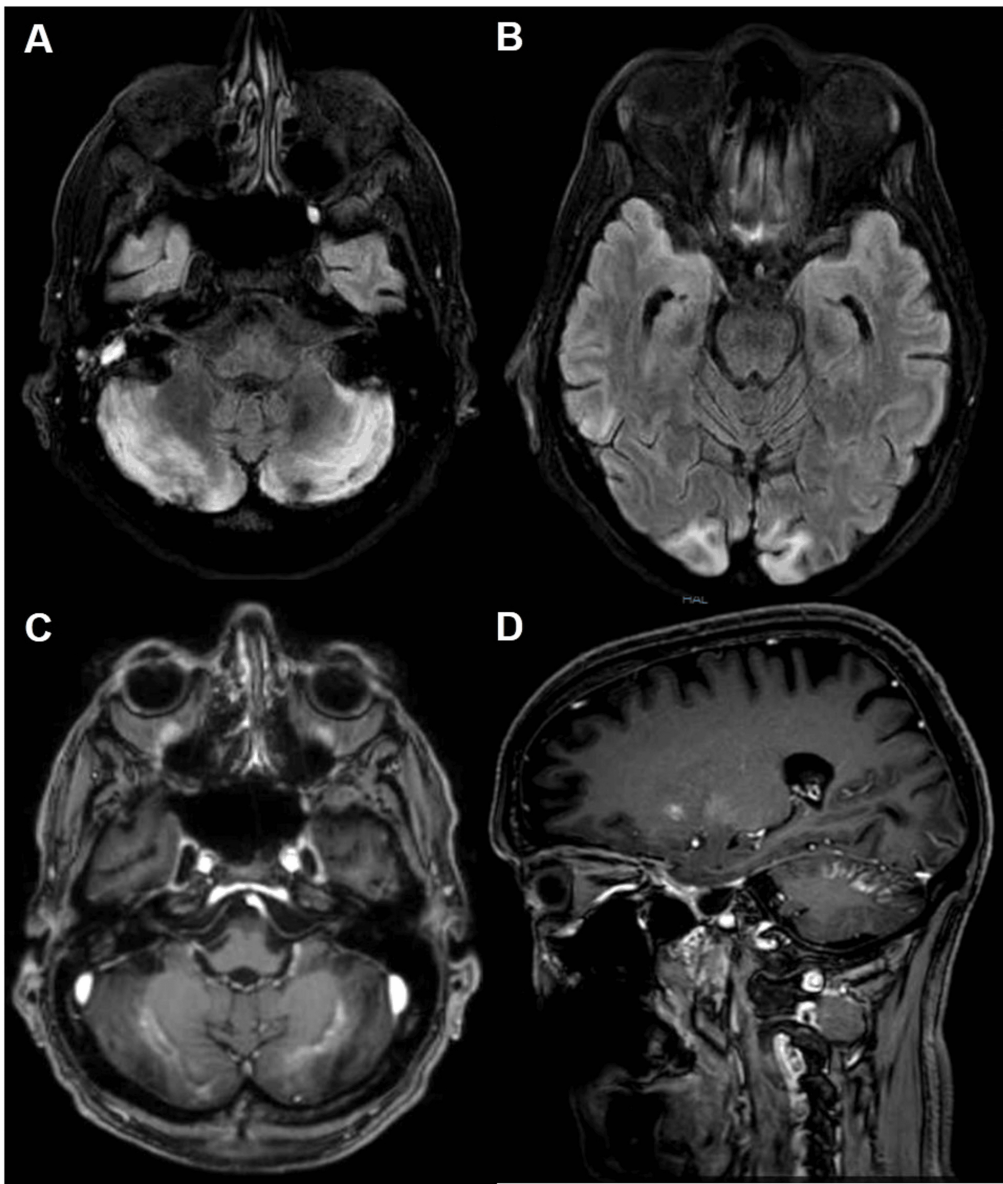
Follow-up brain MRI performed six weeks after admission. (A) T2w FLAIR axial images at the level of the medulla oblongata and the pons demonstrate persistent bilateral hyperintensities of the cerebellar hemispheres (B) with newly developed symmetric hyperintense lesions in the bilateral occipital poles. (C, D) Corresponding post-contrast T1-weighted images (axial and parasagittal) show pathological contrast enhancement within the cerebellar hemispheres and the occipital cortex, indicating ongoing blood-brain barrier disruption.
